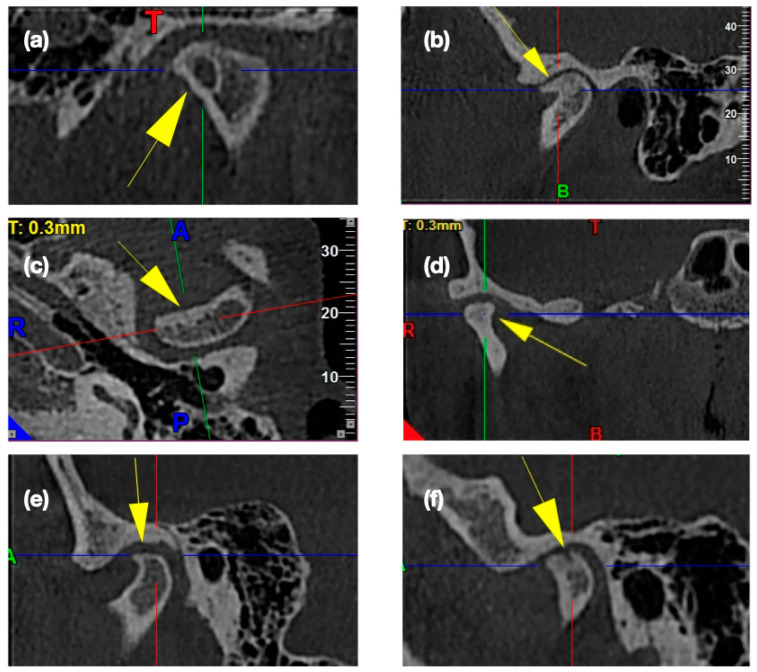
Osteoarthritic changes in the temporomandibular joint cone-beam computed tomography (CBCT) images: (a) Subcortical cyst; (b) Osteophyte; (c) Subcortical sclerosis; (d) Generalized sclerosis; (e) Articular surface flattening; (f) Erosion.
